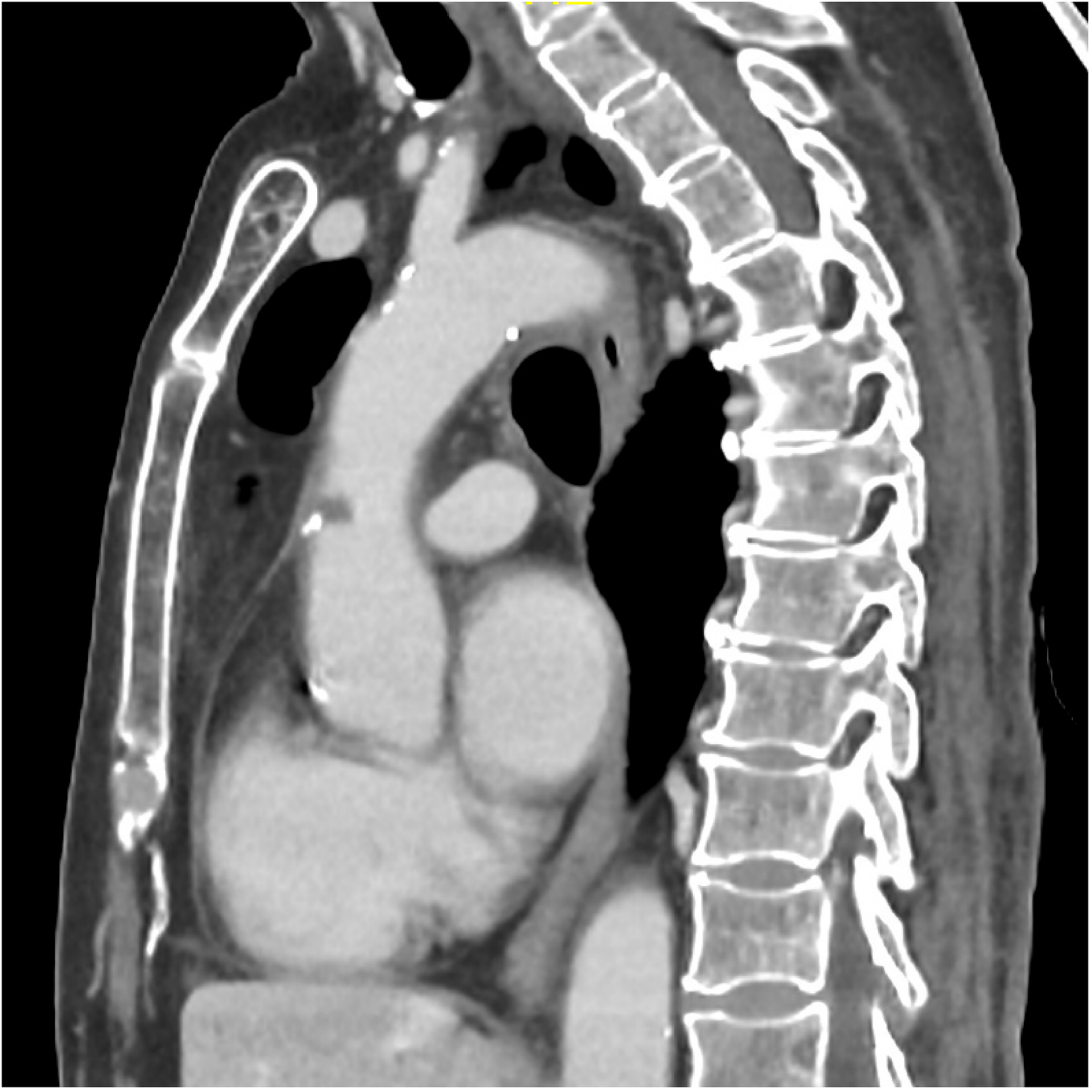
Sagittal CT-scan plane showing the 8 mm thrombus in the ascending aorta.
